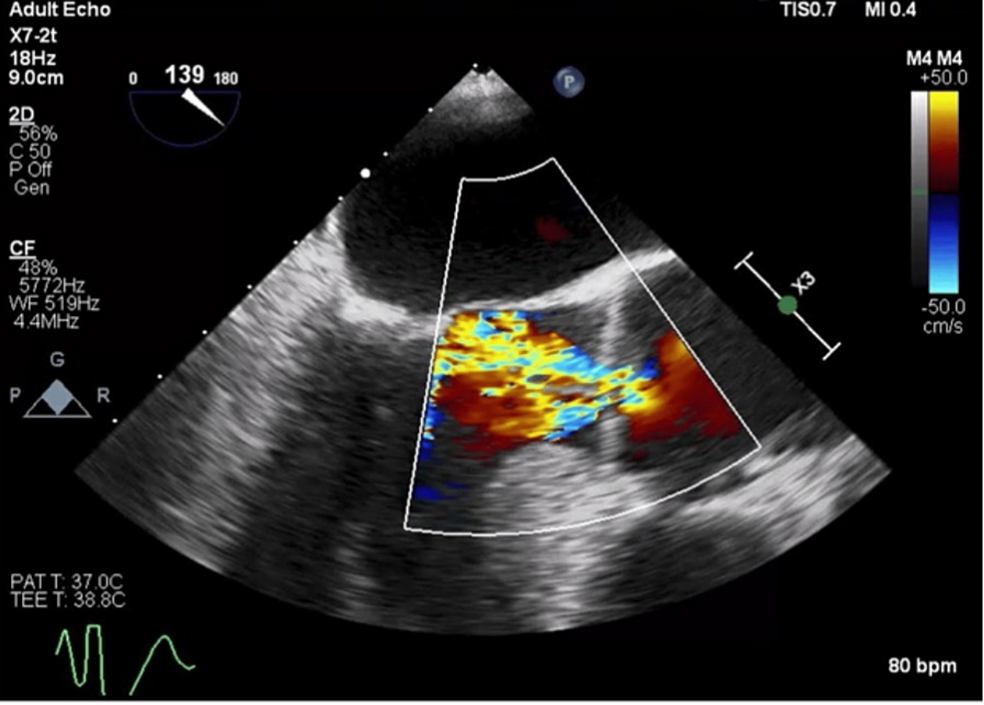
New aortic insufficiency New aortic insufficiency was found on intraoperative TTE prior to CABG and aortic valve repair and replacement. TTE - transesophageal echocardiogram; CABG - coronary artery bypass graft surgery
